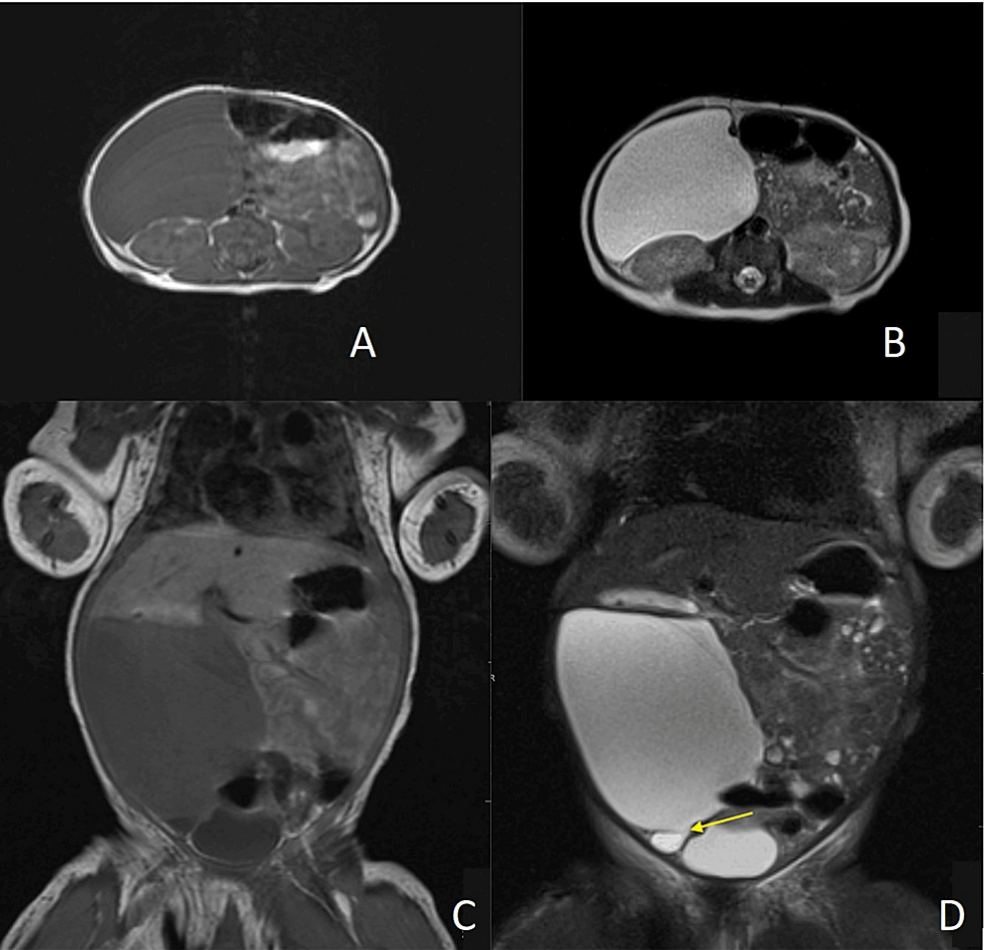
Axial and coronal T1-weighted (A and C) and T2-weighted (B and D) magnetic resonance images of the abdomen. The images demonstrate a large right hemiabdomen hypointense T1/hyperintense T2 mass, compatible with a simple cyst. At the inferior margin of the cyst, the mass was seen to be connected with another cyst, which was felt to be possibly due to an ovarian origin (arrow; D). Significant mass effect on the inferior liver as well as displacement of the bowel loops to the left is noted.
